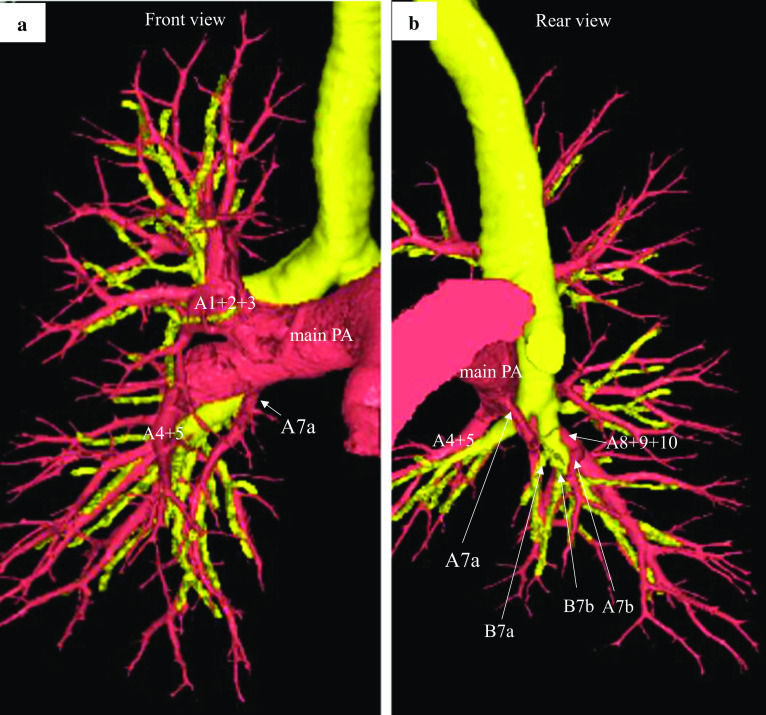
Three-dimensional computed tomography angiography and bronchography images. a Front view. b Rear view. Reconstructed images of arteries (red), veins (blue), bronchi (yellow), and tumor (green). *A* artery; *B* bronchus; *V* vein
